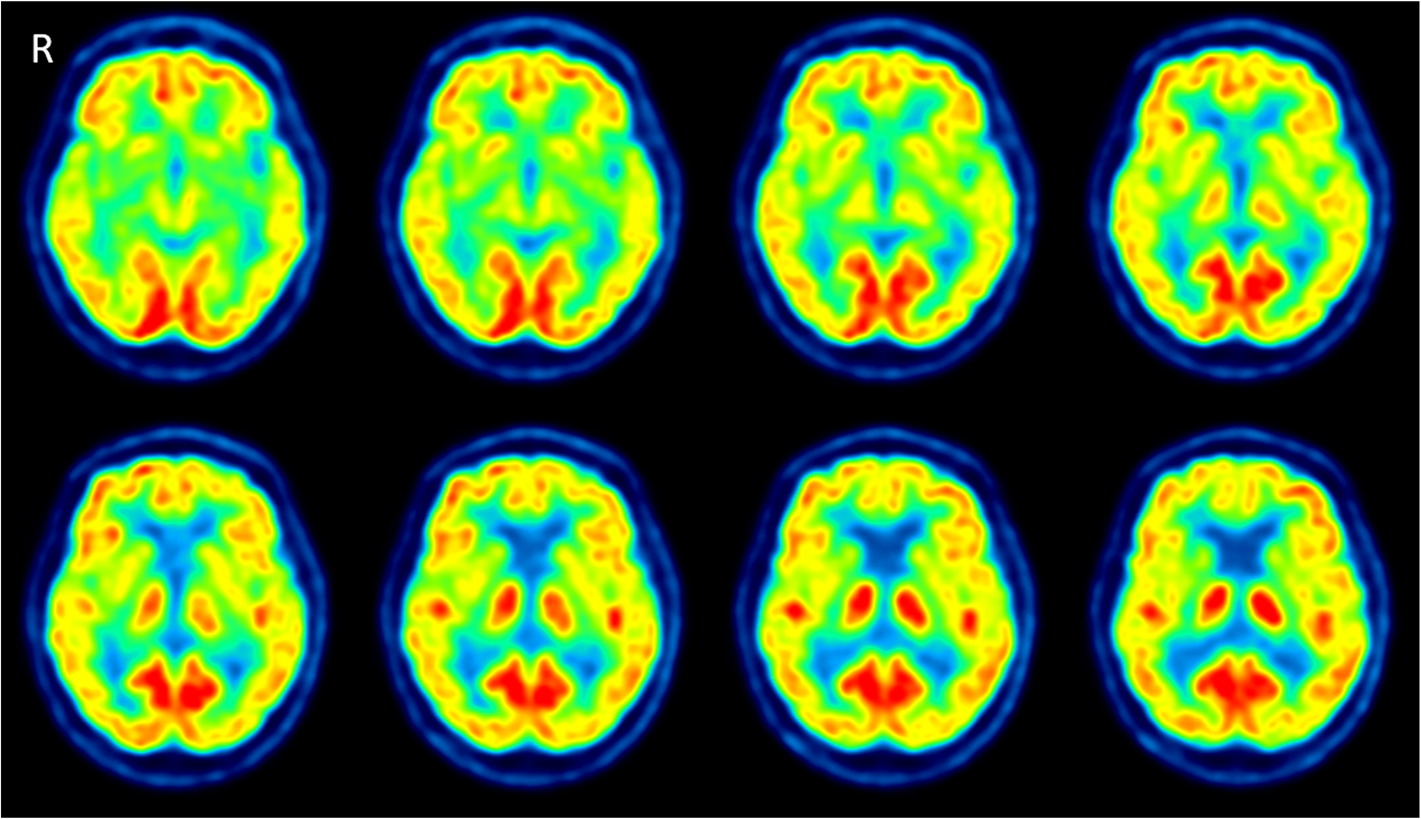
Axial images of ^18^F-FDG PET/CT scan demonstrating the severed striatal hypometabolism
